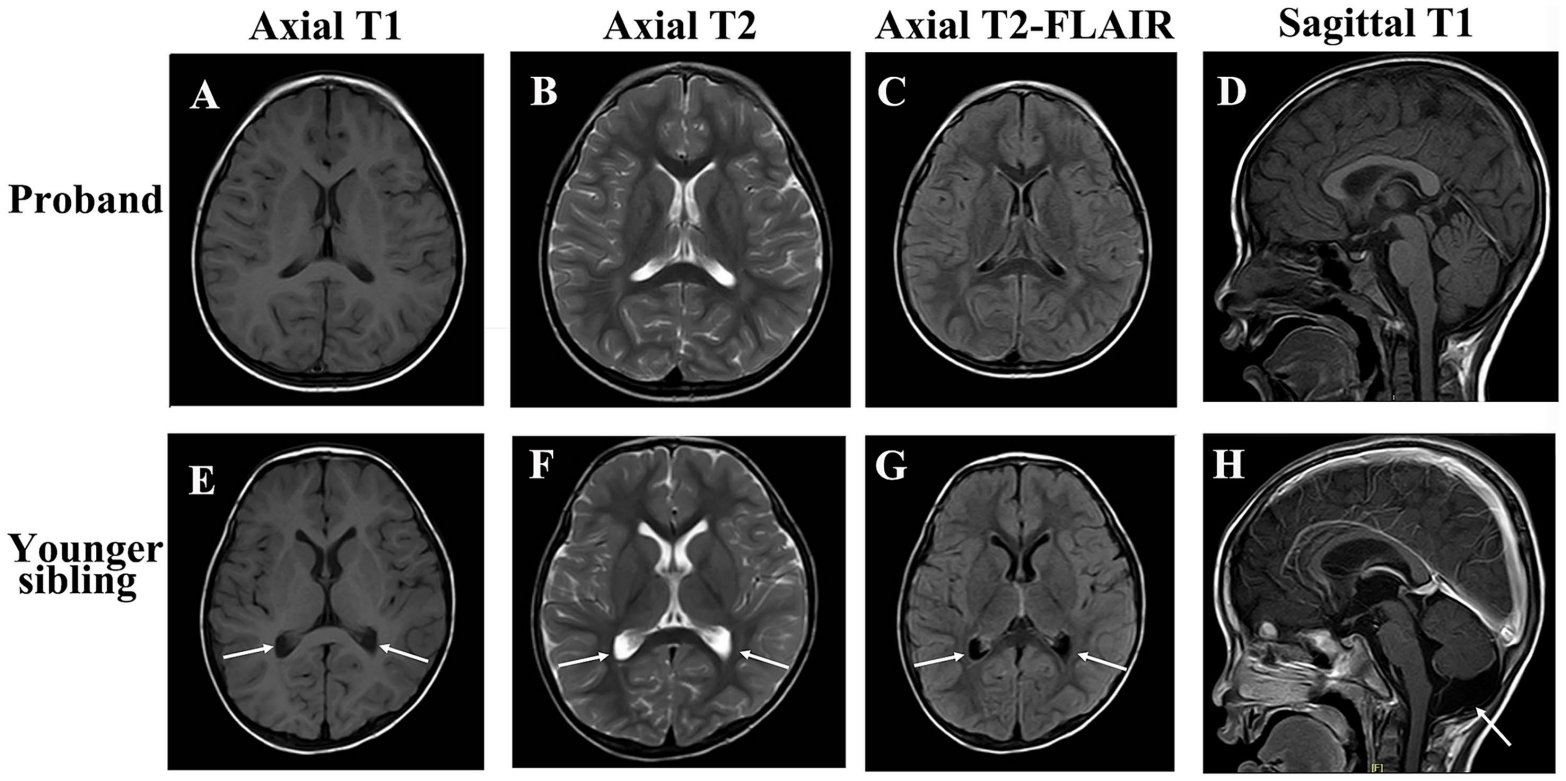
Brain MRI of the patients. Brain MRI of the proband: (A) Axial T1-weighted image; (B) Axial T2-weighted image; (C) Axial T2-weighted FLAIR image. (D) Sagittal T1-weighted No structural abnormalities were observed. Brain MRI of the proband’s younger brother: (E) Axial T1-weighted image; (F) Axial T2-weighted image; (G) Axial T2-weighted FLAIR image. (H) H Sagittal T1 post-contrast; Mild lateral ventricular enlargement and a prominent cisterna magna were noted. Arrowheads in (E–G) indicate mild lateral ventricular enlargement; the arrow in (H) highlights a prominent cisterna magna.
